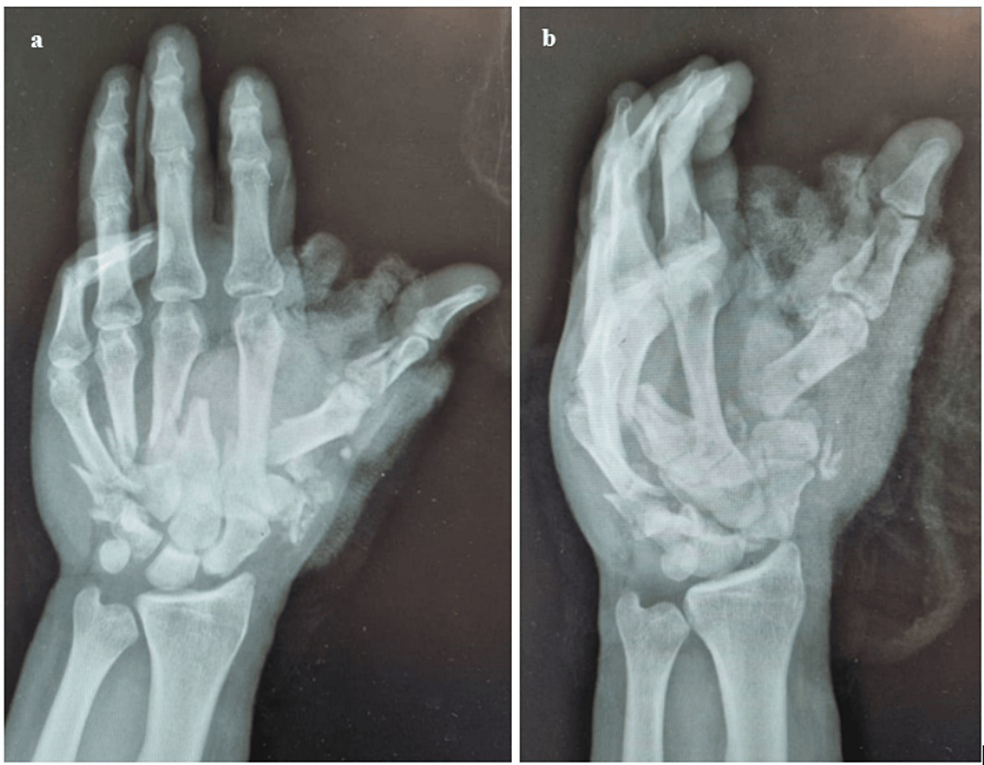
Radiographs of the left hand and wrist revealed a comminuted fracture of the first to fifth metacarpal bones along with carpal row dissociation a) Left-hand radiograph (posteroanterior view). b) Left-hand radiograph (oblique view).
